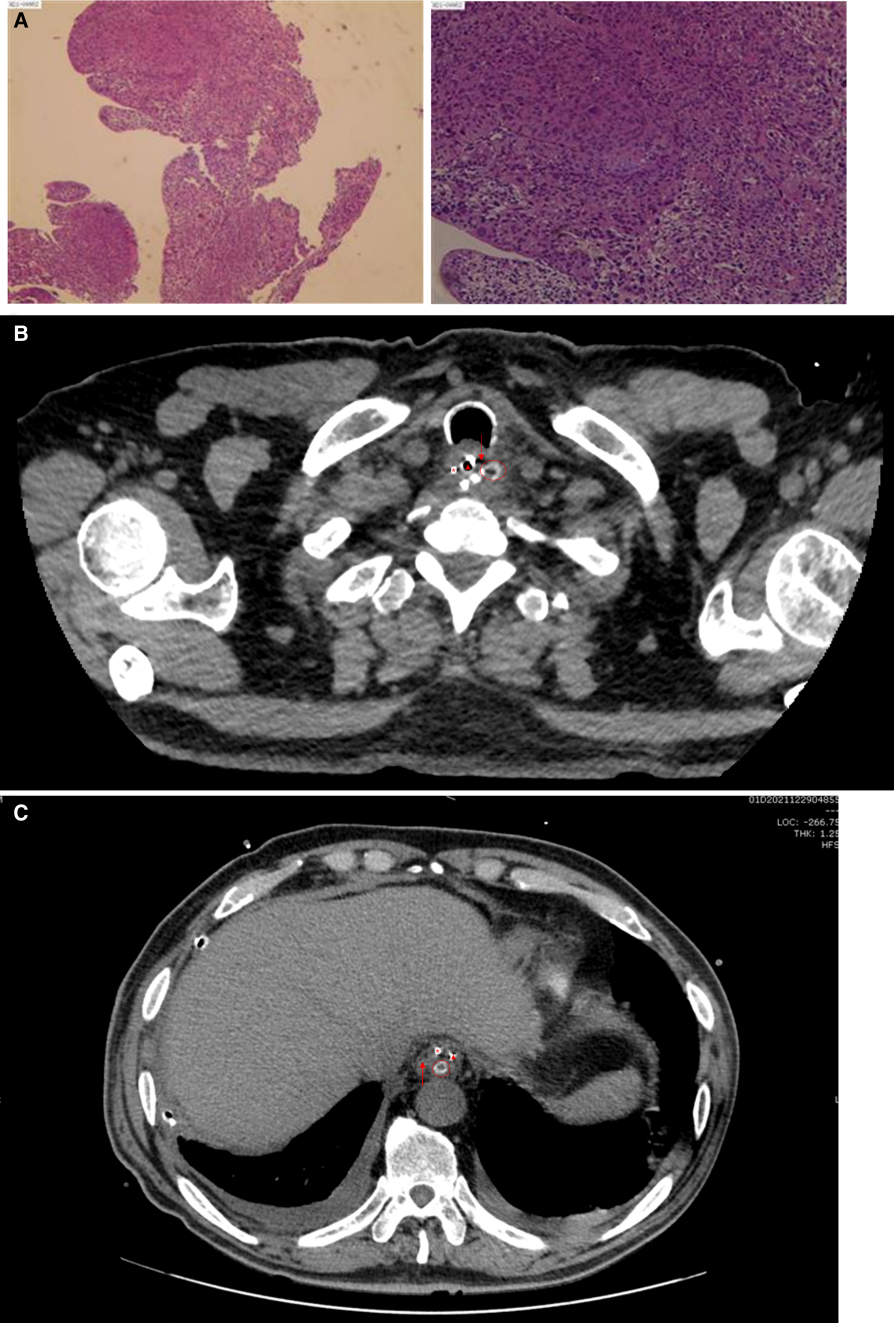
(A) Preoperative pathological pictures of the patient; (B) CT image on POD 7. Images showed that the drainage tube was located outside the esophageal cavity; (C) CT image on POD 37 showed that the cervical drainage tube went deep into the stomach. ○, cervical drainage tube; ▴, gastric tube; *, duodenal nutrient tube; ↓, esophageal cavity; ↑, stomach.
